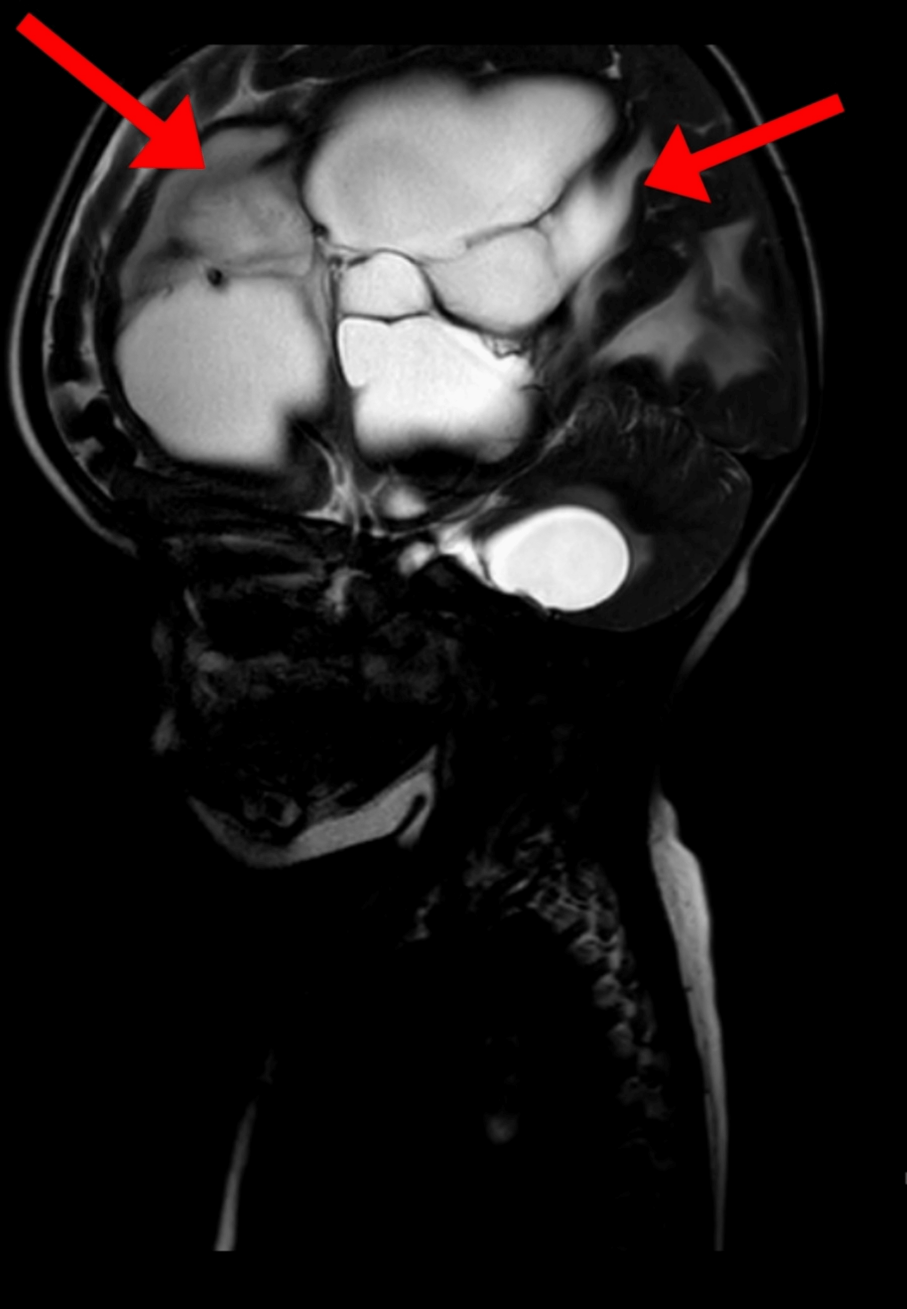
MRI of the brain showing multiple signal intensity loculated cystic lesions noted in bilateral cerebral hemispheres (left frontal, left parietal, bilateral occipital, right temporoparietal) adjacent to the third ventricle and right cerebellar hemisphere adjacent to the fourth ventricle.
